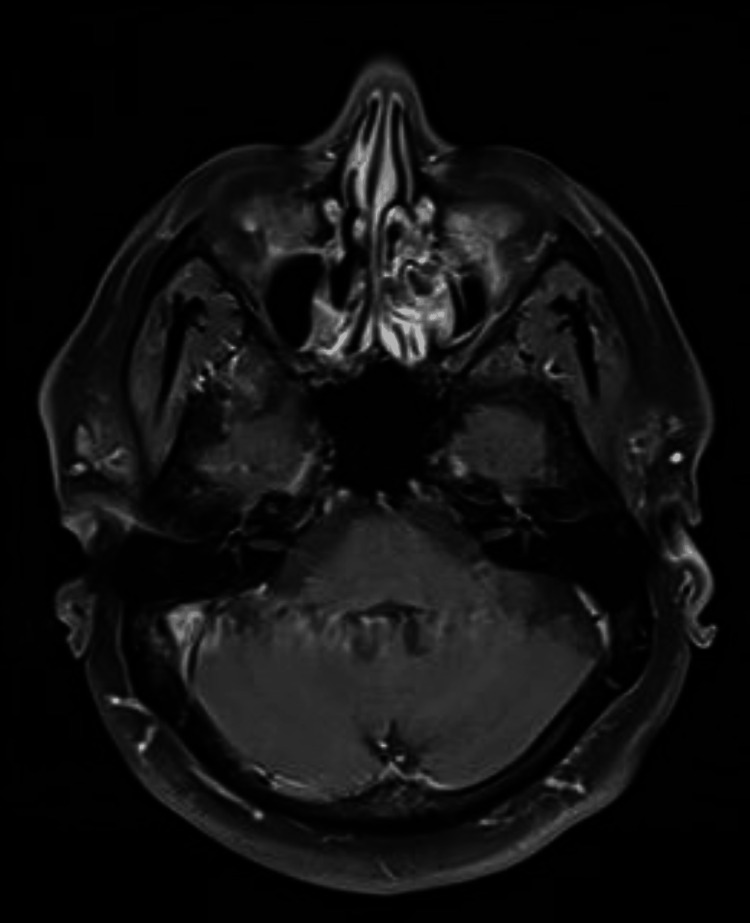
MRI T1-weighted bulbar axial cut
